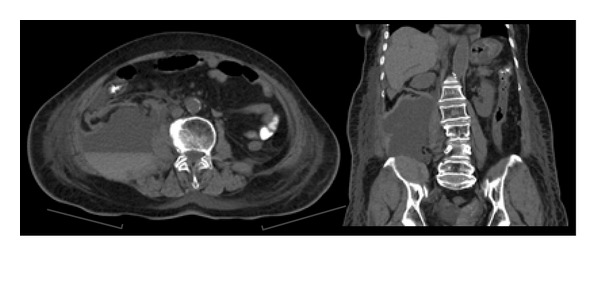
CT retroperitoneal hematoma (Case  1)—a large extraperitoneal hematoma measuring 18.3 × 11.2 cm arising from the posterior surface of lower part of left rectus abdominis muscle and extending into the pelvis causing right sided displacement of pelvic organs.
